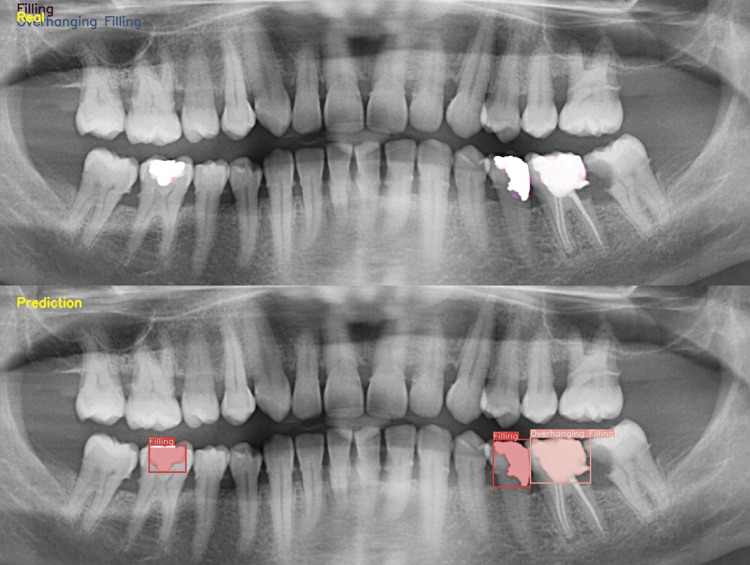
Panoramic radiograph depicting estimated segmentation images, like Figure 2, generated by a real and artificial intelligence model for detection of overhanging and normal fillings in a separate patient.
